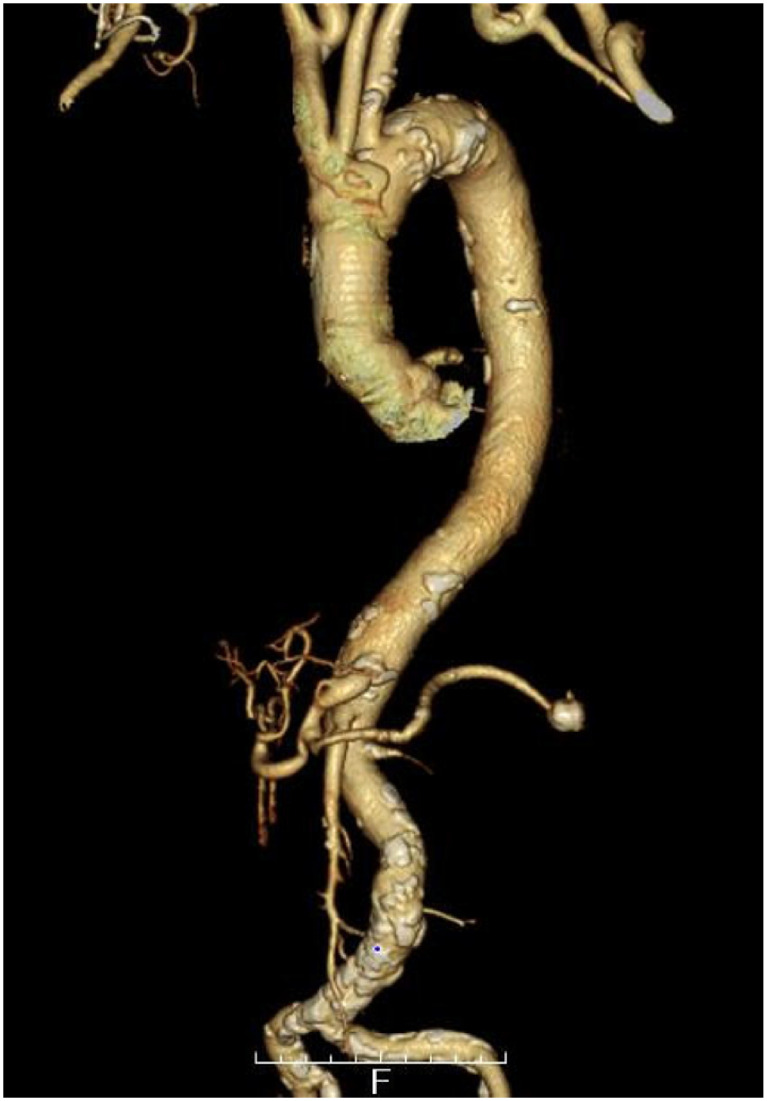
Fig. 3 Postoperative contrast-enhanced computed tomography. There is no residual dissection, and both the celiac and superior mesenteric artery are well enhanced.
